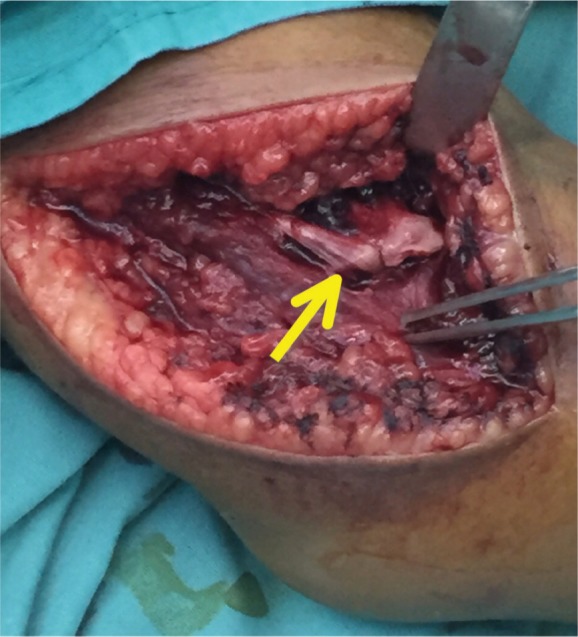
Radiograph showing fracture of the supracondyle of right humerus. Yellow arrow showing radial nerve.
